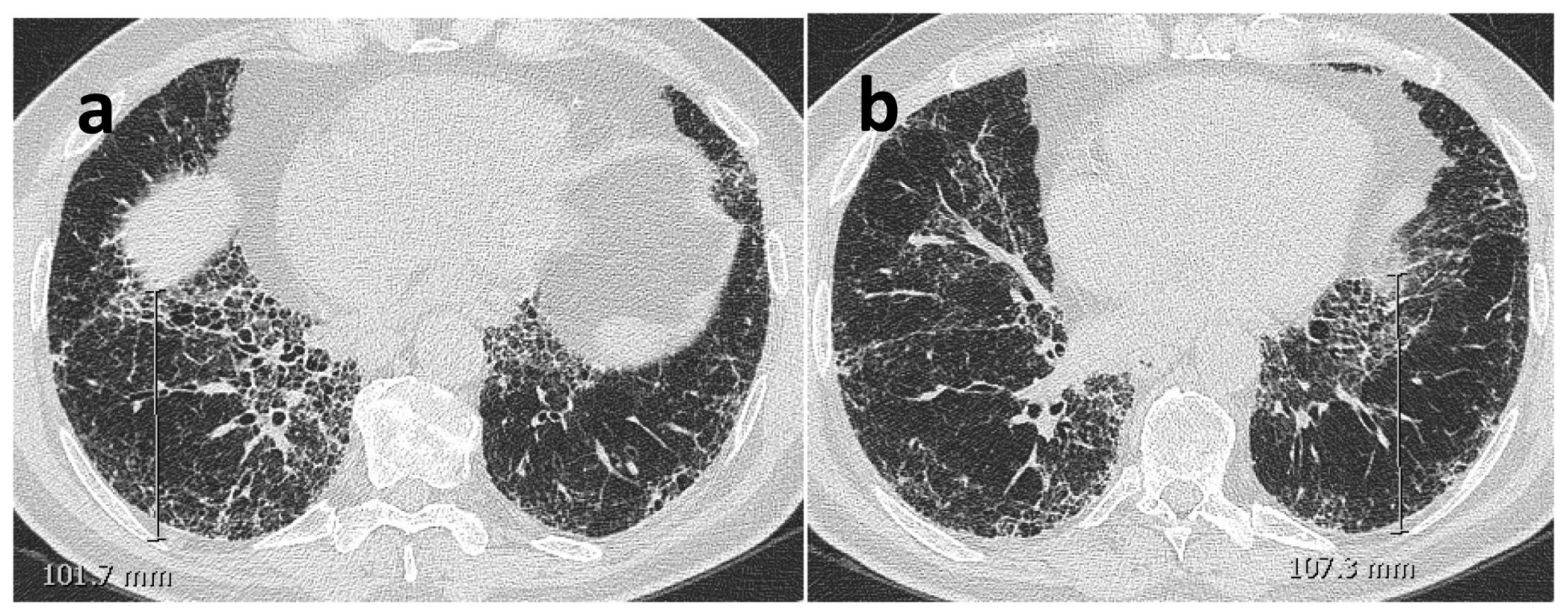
Axial non-contrast enhanced CT images of a 71-year-old male with IPF. The distance between where the fissure meets the diaphragm to the most posterior aspect of the lung was measured on the right (a). The measurement was repeated on the left lung (b) and mean value was calculated to obtain the oblique fissure posterior retraction distance (OFPRD).
